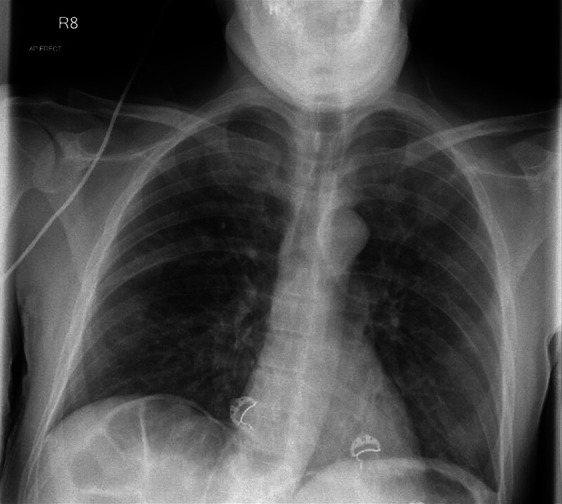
Chest radiograph performed at the time of initial oxygen requirement. Formally reported as showing minimal changes.
